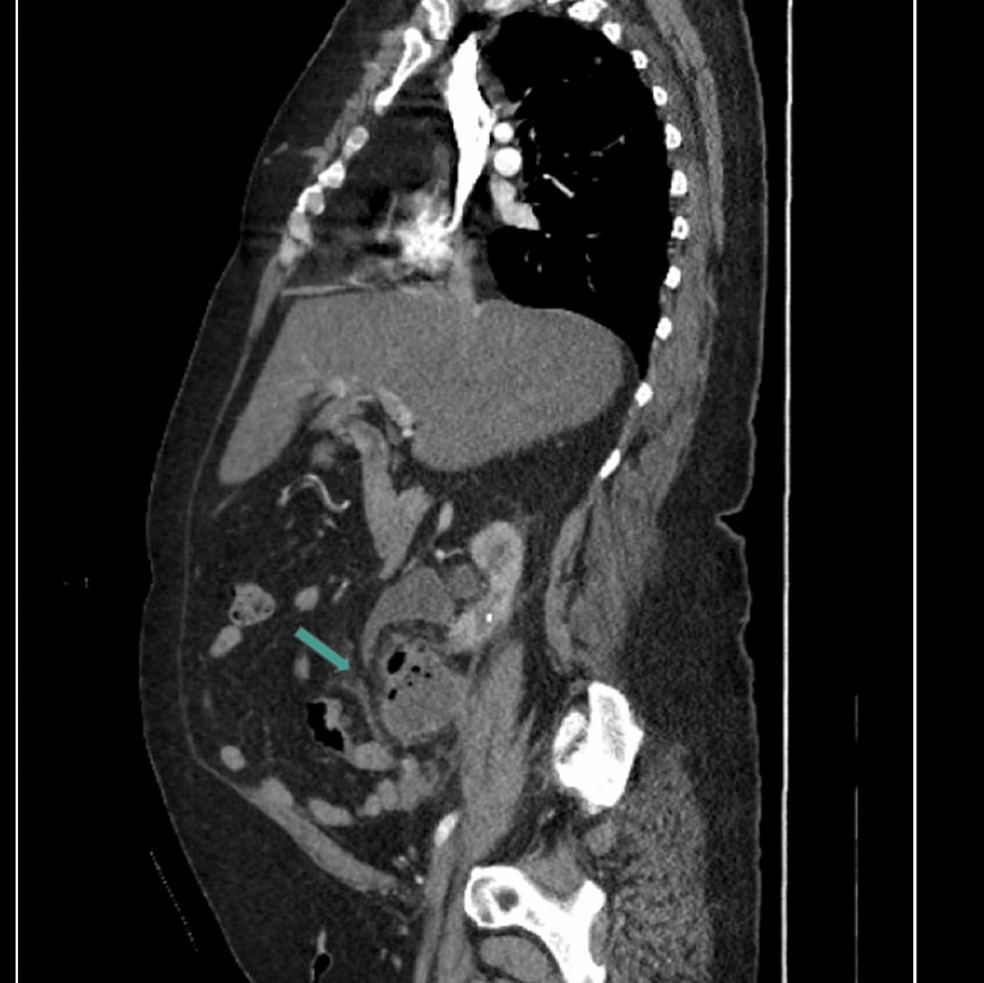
CT sagittal view representing the peri-ureteral fluid and gas-filled lesion, consistent with abscess CT: computed tomography
